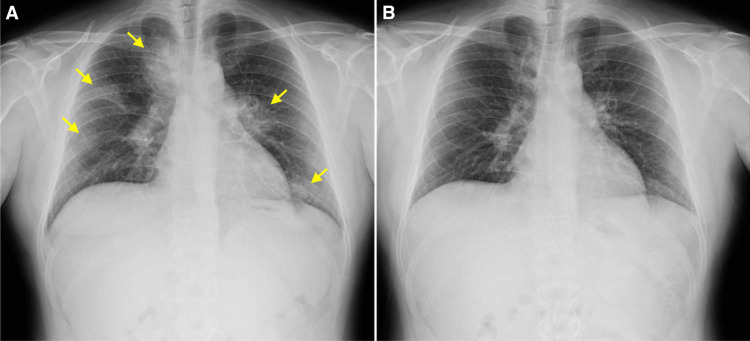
Chest x-ray findings before and after steroid therapy. (A) Chest x-ray taken prior to steroid therapy shows multiple consolidations (arrows) consistent with pulmonary sarcoidosis. (B) Chest x-ray shows multiple consolidations resolved after steroid therapy.
